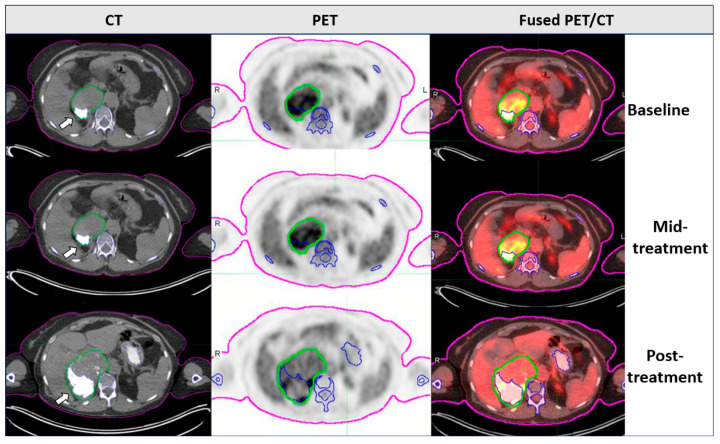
Longitudinal axial CT, PET, and fused PET/CT images demonstrating the presence of ossification (white arrow) in the abdominal DDLPS tumor over the course of treatment. The ossification increased in volume over time; however, the ^18^F-FDG uptake decreased as a treatment response. Since the ossification was invading the tumor volume, this patient was excluded from semiquantitative PET/CT analysis. Delineation color legend: pink = body contour; blue = bone, green = tumor contour.
